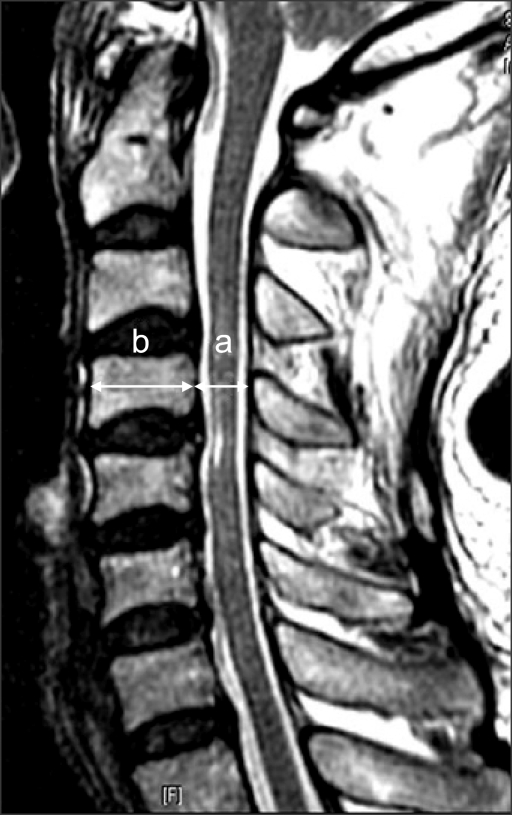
On the MR image, the sagittal diameters of the CSF column (a) and vertebral body (b) are measured at the midvertebral level on T2 sagittal images. The CSF column/vertebral body ratio is determined using the formula a/b.
